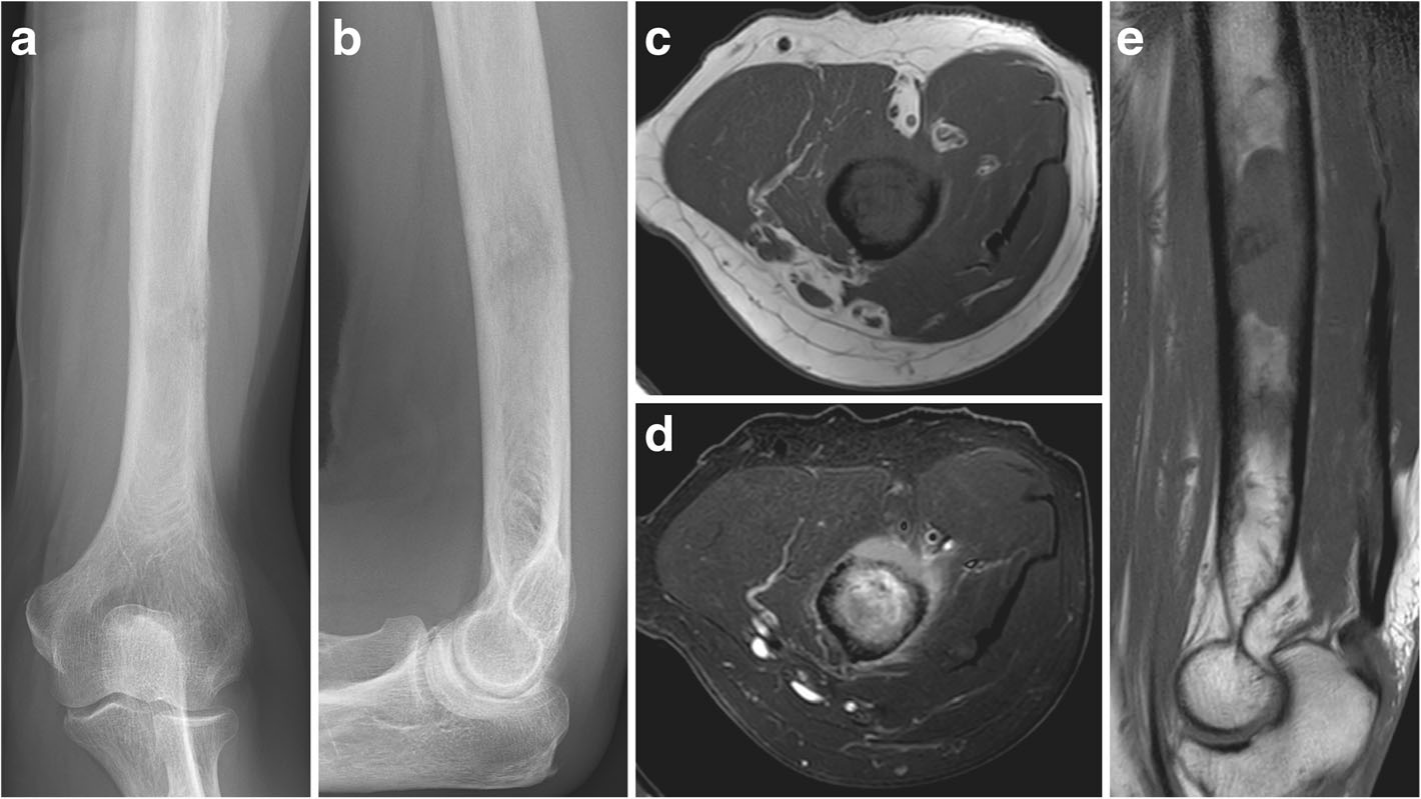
Initial radiographs of the lytic lesion in the distal humeral diaphysis (a, b). MRI with axial T1 (c) and STIR (d) sequences of the lytic lesion with significant cortical erosion and perifocal oedema. The sagittal T1 sequence (e) shows the longitudinal extent of the lesion
